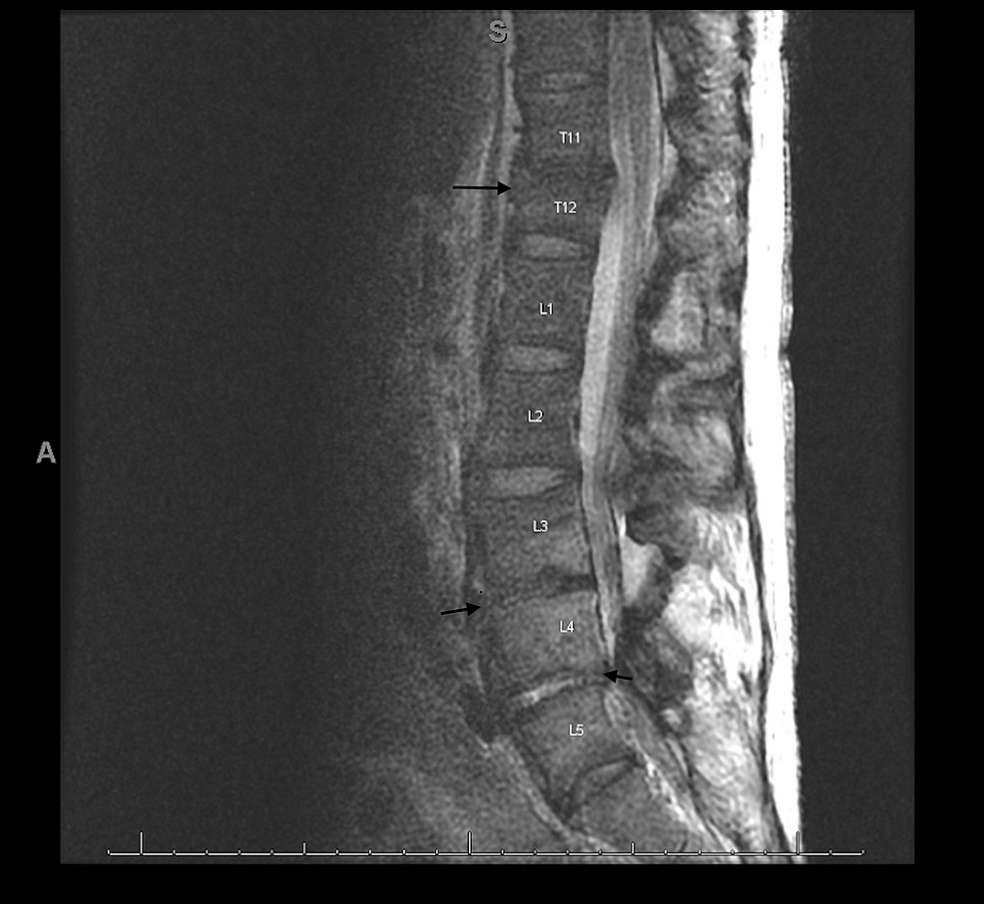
Lumbar spine MRI showing chronic T12 compression deformity, mild spinal stenosis, multifactorial central and left lateral L3/L4 and L4/L5 spinal stenosis, high grade left lateral L4/L5 foraminal stenosis.
